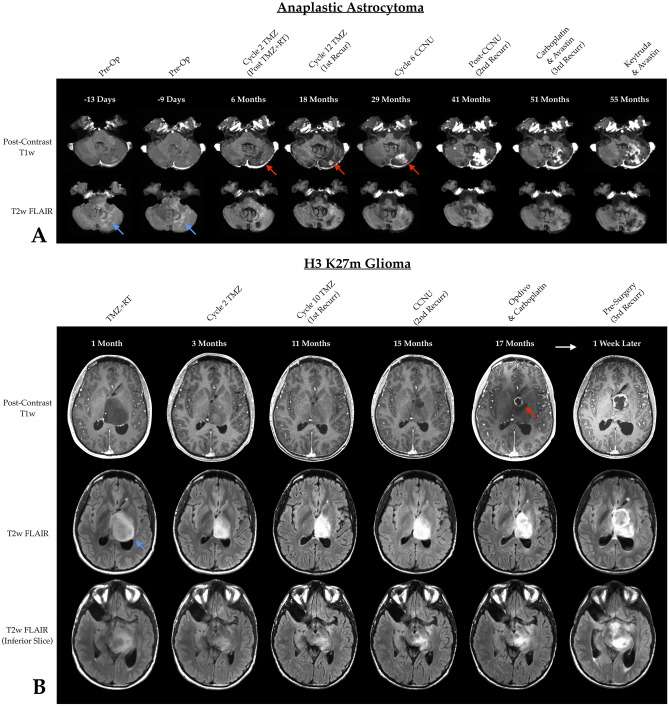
A A 64-year-old female patient with a cerebellar grade 3 anaplastic astrocytoma (AA) showing serial post-contrast T1-weighted (top row) and T2-weighted FLAIR images (bottom row). The lesion started out non-enhancing and only (blue arrows), but over time developed both enhancing (red arrows) and non-enhancing tumor growth. B A 22-year-old male patient with an H3 K27m mutation showing serial post-contrast T1-weighted (top row) and T2-weighted FLAIR images (middle row). Also showed is T2-weighted FLAIR of an inferior slice demonstrating tumor growth. Similar to (A), this patient demonstrated early non-enhancing tumor (blue arrows) that later developed enhancing disease (red arrow), both of which continue to grow
